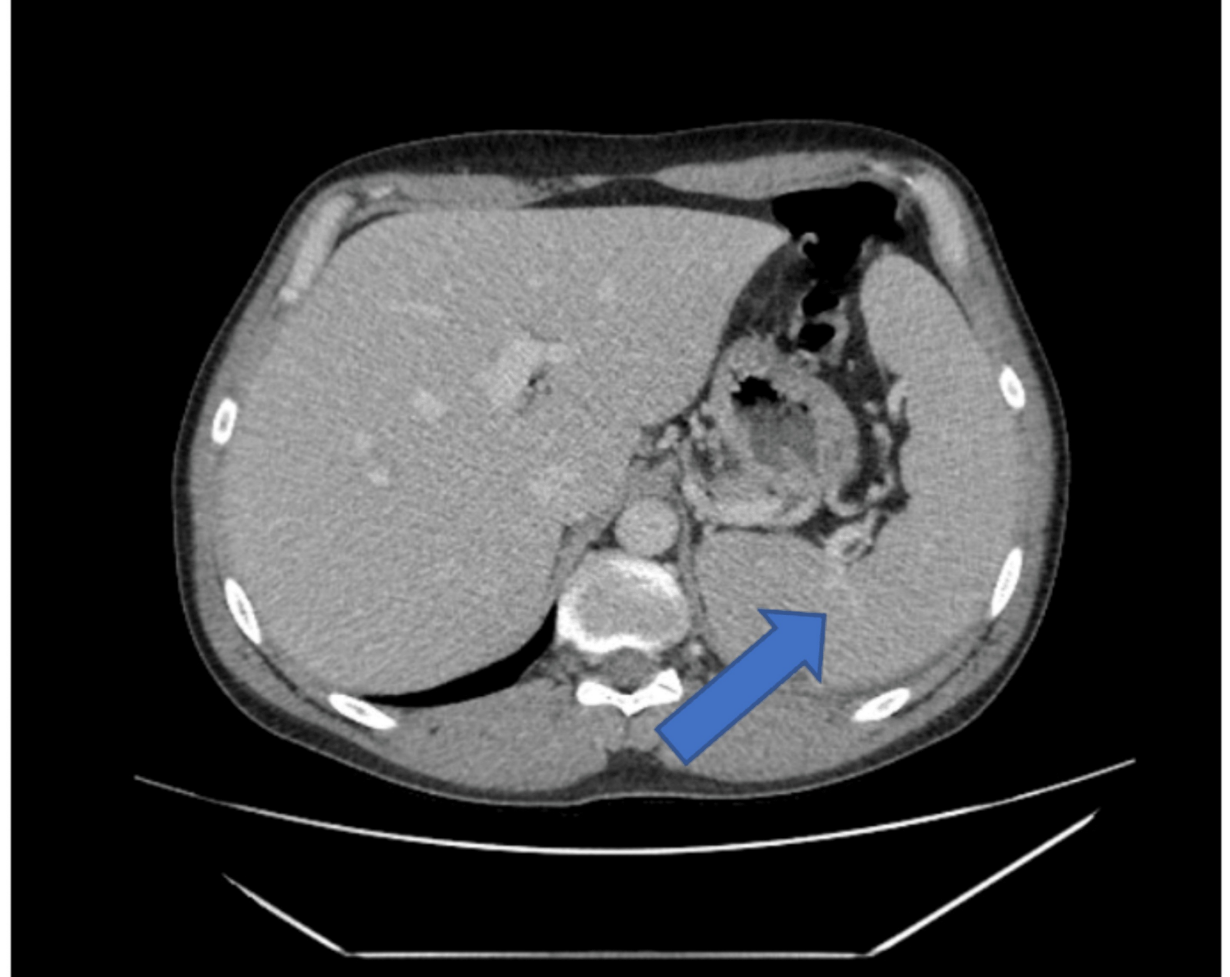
Computed tomography of the abdomen and pelvis demonstrating splenomegaly (blue arrow)
